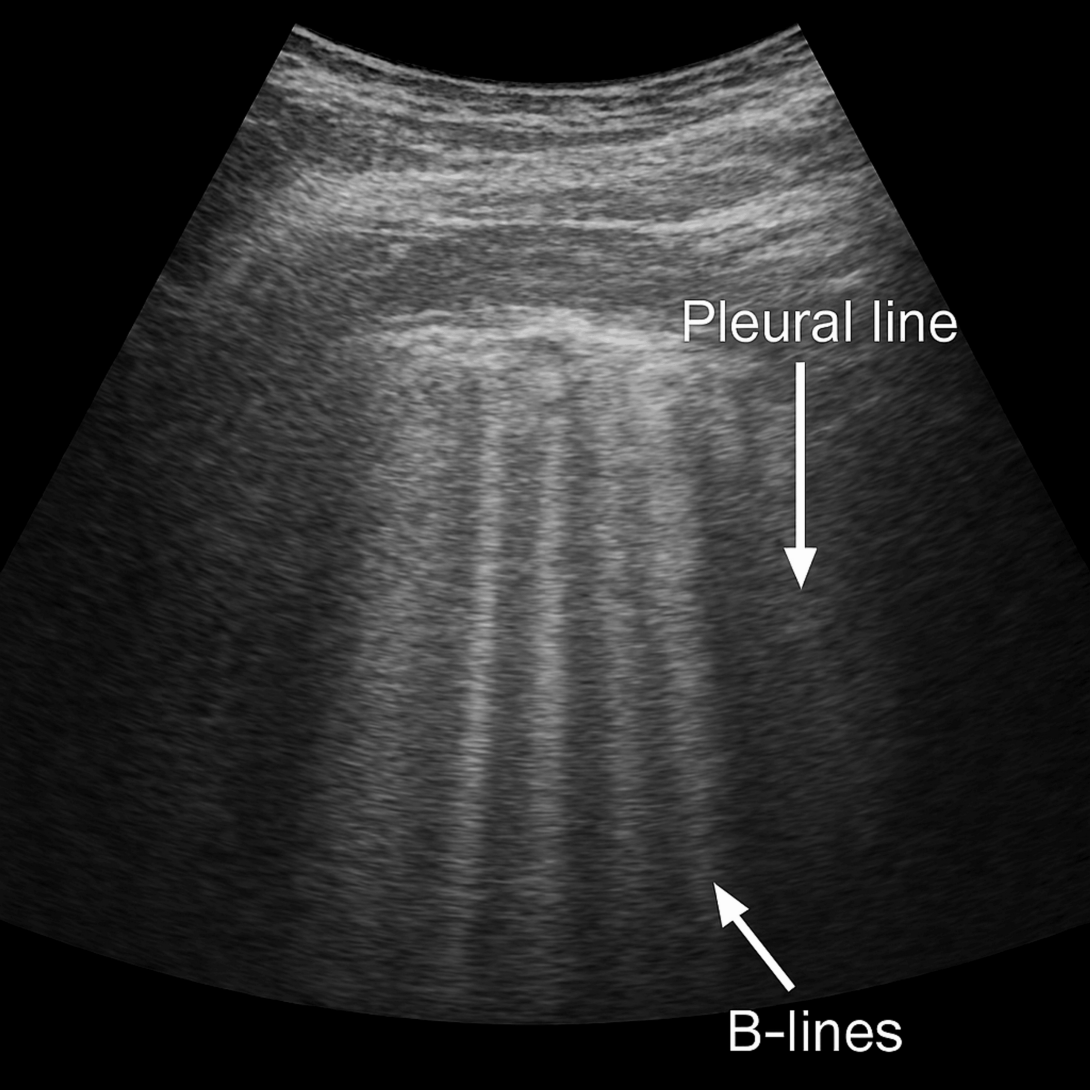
Lung ultrasonography demonstrating more than three B-lines (comet-tail artifacts) in a single intercostal space, indicative of pulmonary edema
